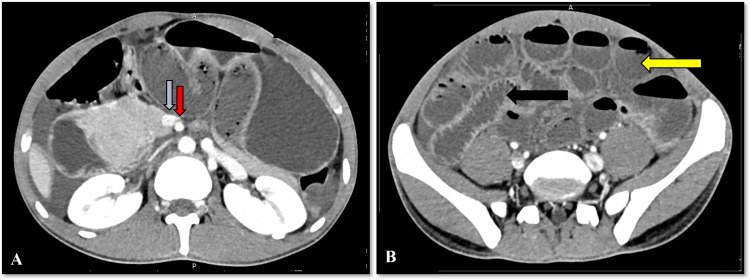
Axis of superior mesenteric vessels and gut malrotation Axial sections of the CECT abdomen show the maintained axis of the superior mesenteric artery (red arrow) and superior mesenteric vein (blue arrow). Jejunal loops are seen on the right side of the peritoneal cavity (black arrow) and ileal loops (yellow arrow) are seen on the left side of the peritoneal cavity. CECT: contrast-enhanced computed tomography
